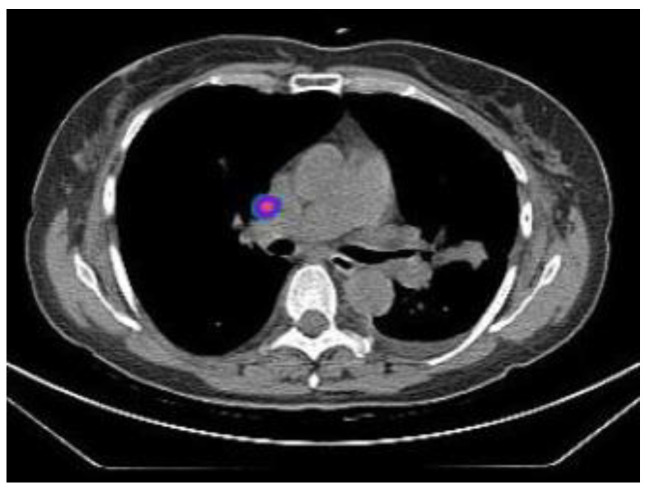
SPECT scan identification of a hilar lymph node as a sentinel lymph node.
